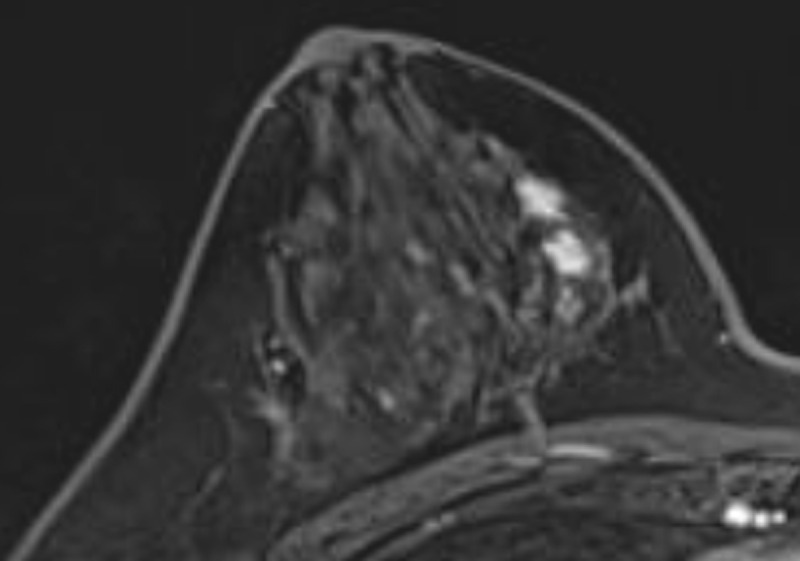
An irregular mass with homogeneous enhancement of the right breast on DCE-MRI.
